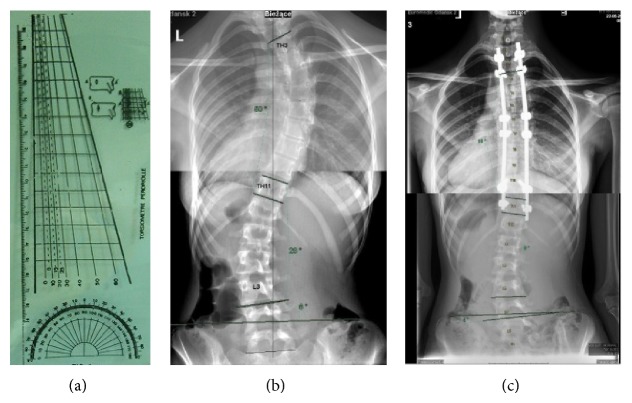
(a) Perdriolle torsionmeter, (b) pre- and (c) postcorrectional Cobb angle measurement on plain radiographs.
